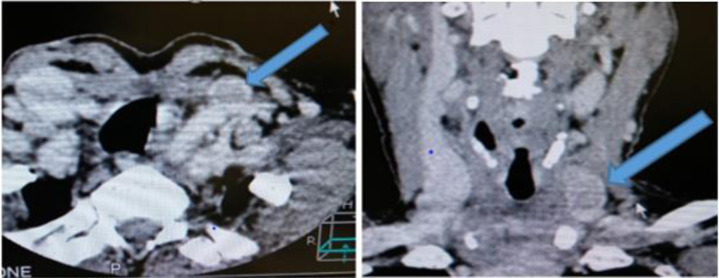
Contrast-enhanced CT scan showing a left IJV intraluminal lesion with a surrounding rim of contrast medium ‘the ring sign’ (arrows).
